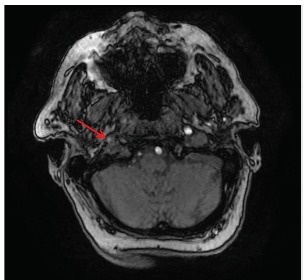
Computed tomography angiography of the neck showing a calcified flow restricting lesion (red arrow) at the origin of the innominate artery.
